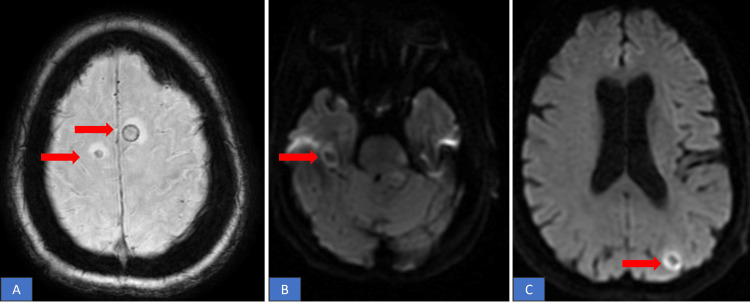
(a,b,c) MRI brain showing multiple enhancing foci noted bilaterally consistent with brain metastases.
